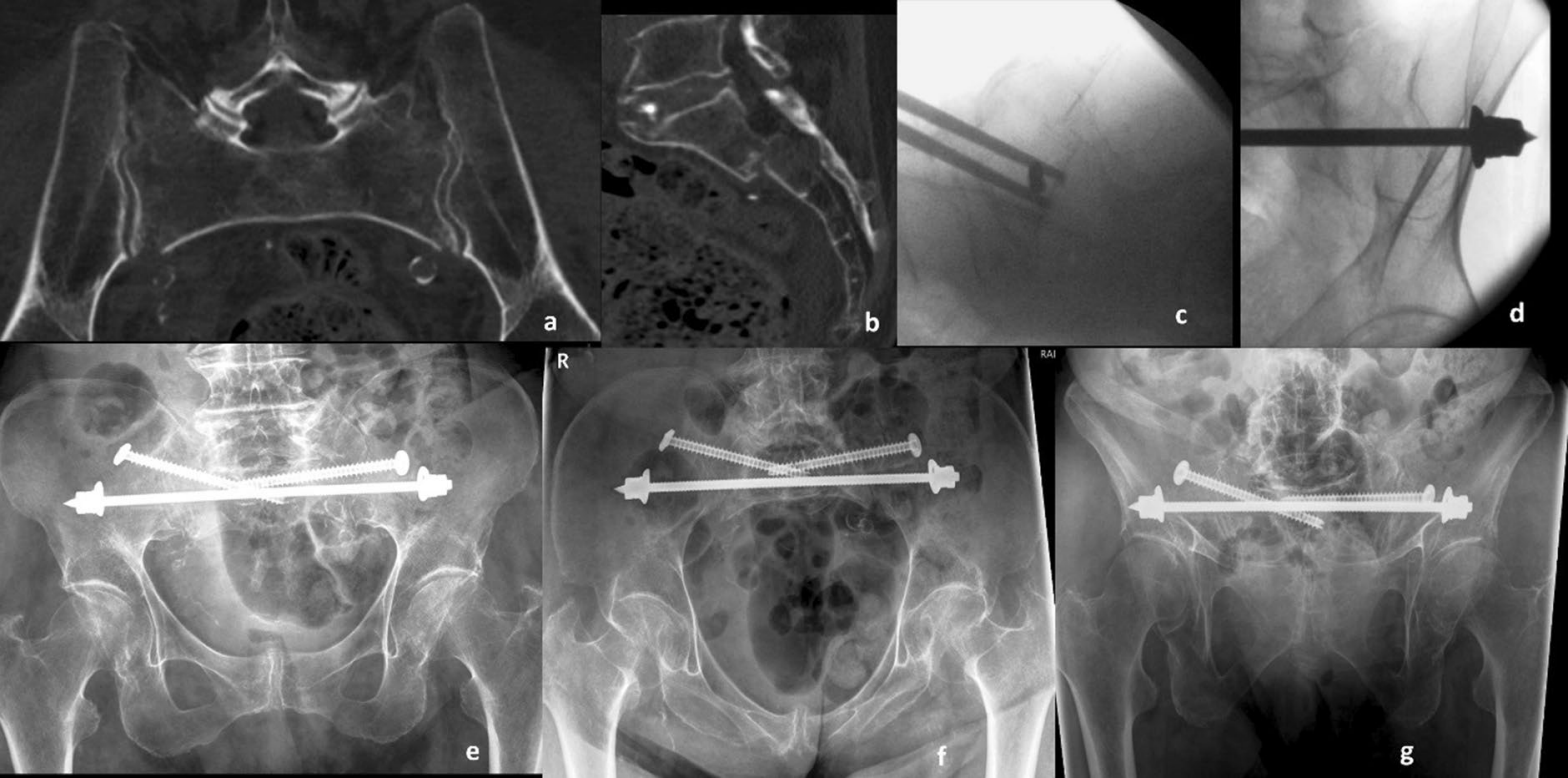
92 year old female patient presenting 1 week after fall from standing height with immobilizing pain at lower lumbar spine. (a) Axial CT demonstrating slightly displacement of bilateral sacral fracture; (b) sagittal CT with displaced transversal fracture at S1/S2 level. The fracture was classified as FFP type IVb. (c) Lateral fluoroscopic image orientated in direction of the patient (bottom anterior, left cranial) showing overlapping of iliocortical densities and ischial notch. The drill guide is placed centrally in S1 corridor caudal to the iliocortical densities. (d) Oblique obturator view to assess the abutment of the washer on the iliac cortex. Postoperative control at discharge, patient is mobile with a walker ((e) anterior–posterior, (f) inlet, and (g) outlet view).
